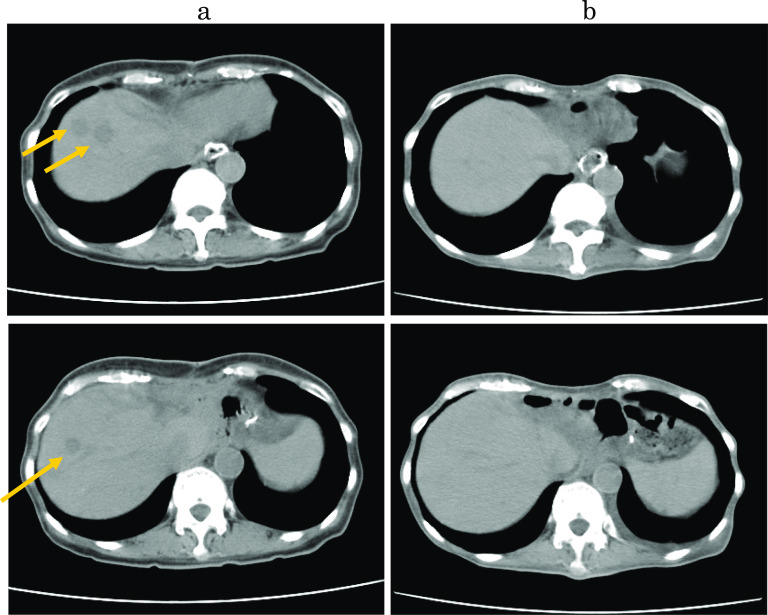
Abdominal CT imaging. a CT scan showed multiple liver metastases in the right lobe of the liver (yellow arrow). b The liver metastases disappeared after the S-1 plus CDDP chemotherapy
